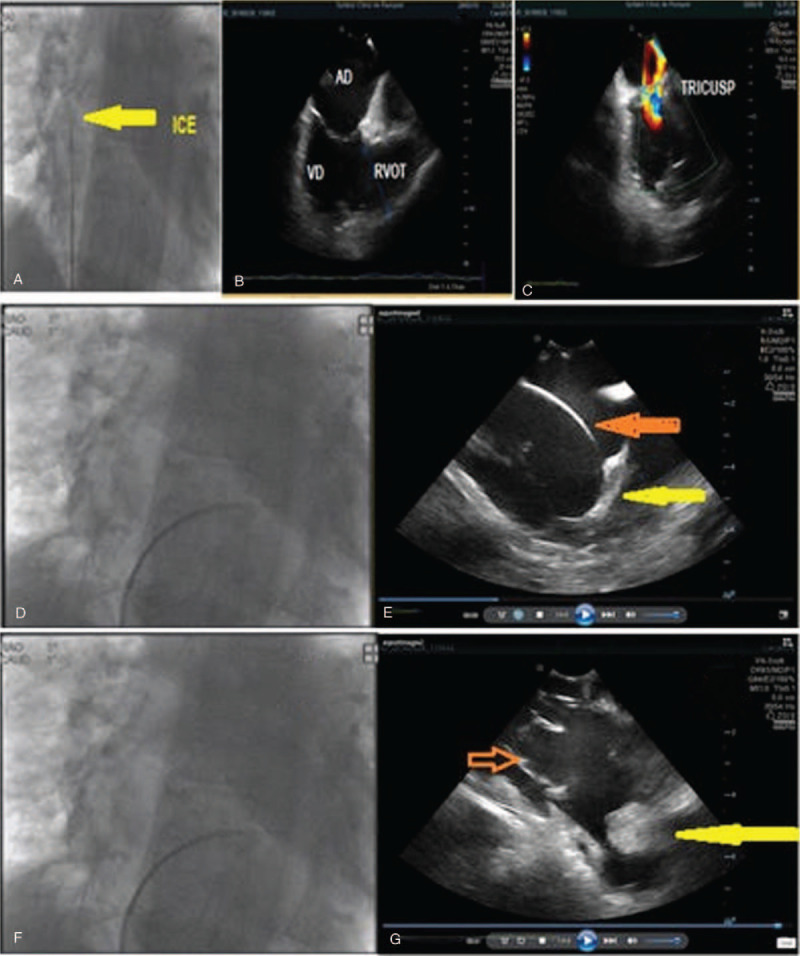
Intracardiac echocardiography using a sectorial probe. (A) Chest X-ray: the ICE probe is inserted in the middle of the right atrium. (B) The RA (44 mm), RV (52 mm), and RVOT (48 mm) are dilated. (C) As the tricuspid ring is dilated, a moderate tricuspid regurgitation is present. (D) Chest X-ray: Intracardiac echography with the probe inserted inside the right ventricle. (E) Near the apex of the right ventricle the moderator band can be visualized (yellow arrow). (F) Chest X-ray: Intracardiac echography with the probe inserted inside the right ventricle. (G) The patient is implanted with an ICD (red arrow) for the secondary prevention of sudden cardiac death as he presented many episodes of ventricular tachycardia. At the apex of the right ventricle a localized aneurysm can be seen with a pediculated thrombus (yellow arrow). ICD = internal cardiac defibrillator, ICE = intracardiac echocardiography, RV = right ventricle, RVOT = right ventricular outflow tract.
